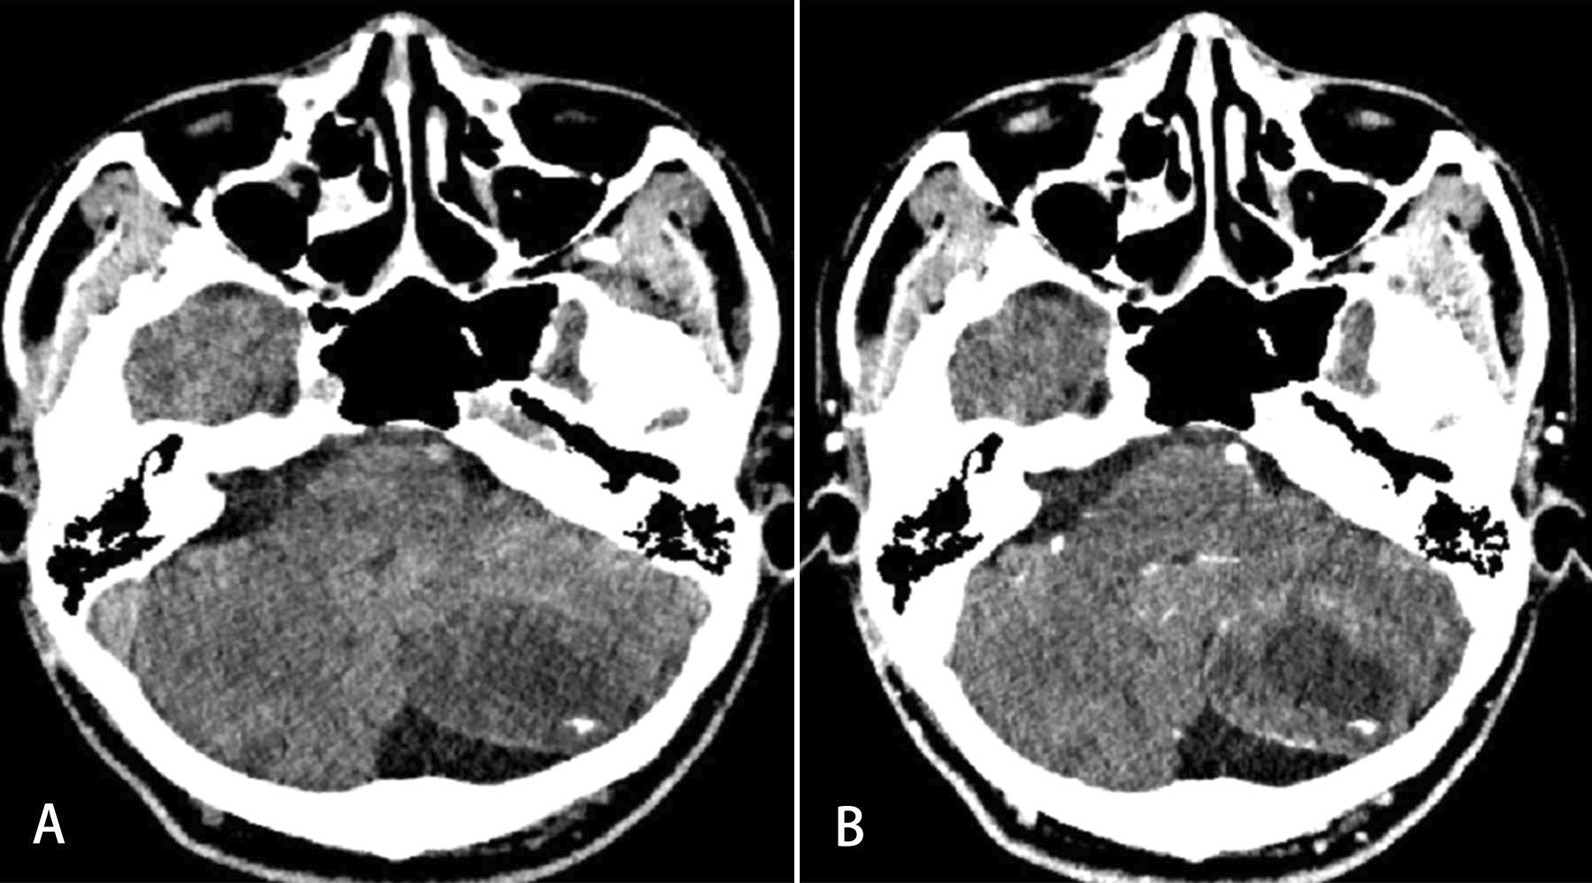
CT findings of a 42-year-old man with intraparenchymal schwannoma. Nodular calcification could be observed along the edge of the solid and cystic mass in the left cerebellar hemisphere (A, B)
